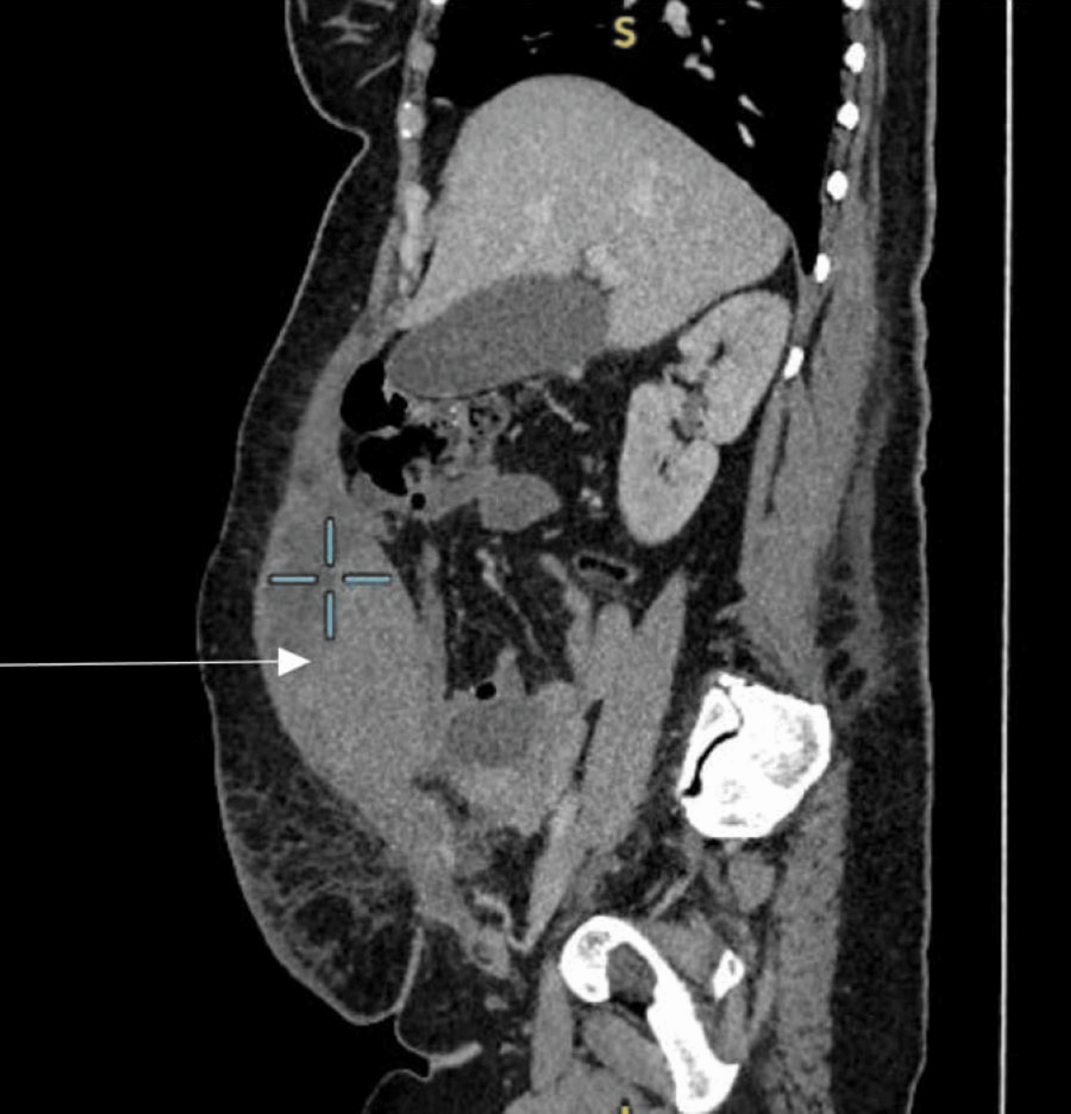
Computed tomography (CT) scan image of the rectus sheath haematoma in sagittal view (arrow indicating the haematoma).
